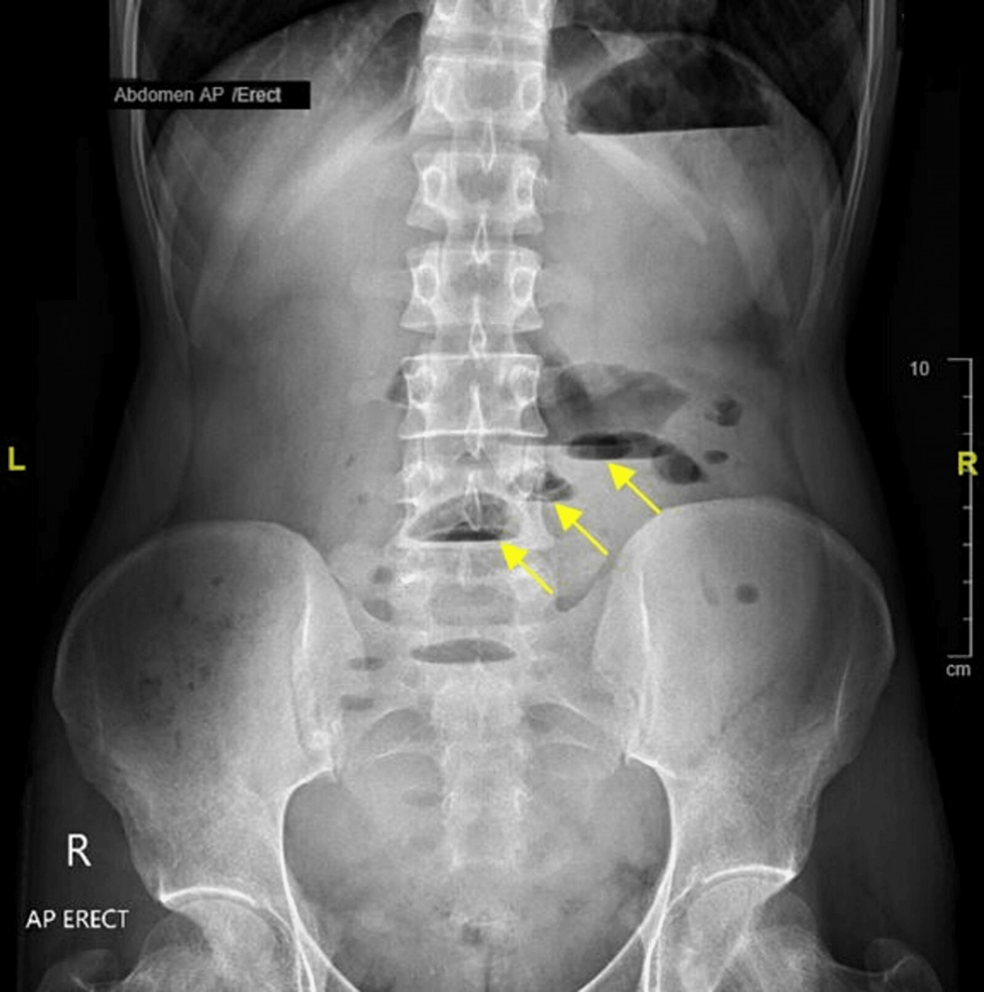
Erect abdominal X-ray at admission showing multiple air-fluid levels (yellow arrows), consistent with the clinical picture of acute small bowel obstruction.
